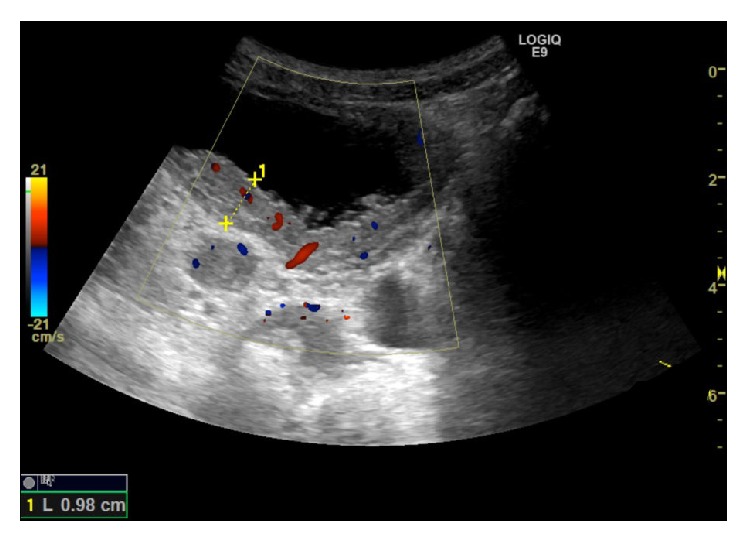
Ultrasound showed irregular bladder wall thickening of up to 1 cm resembling a tumor and Doppler flow within the wall.
